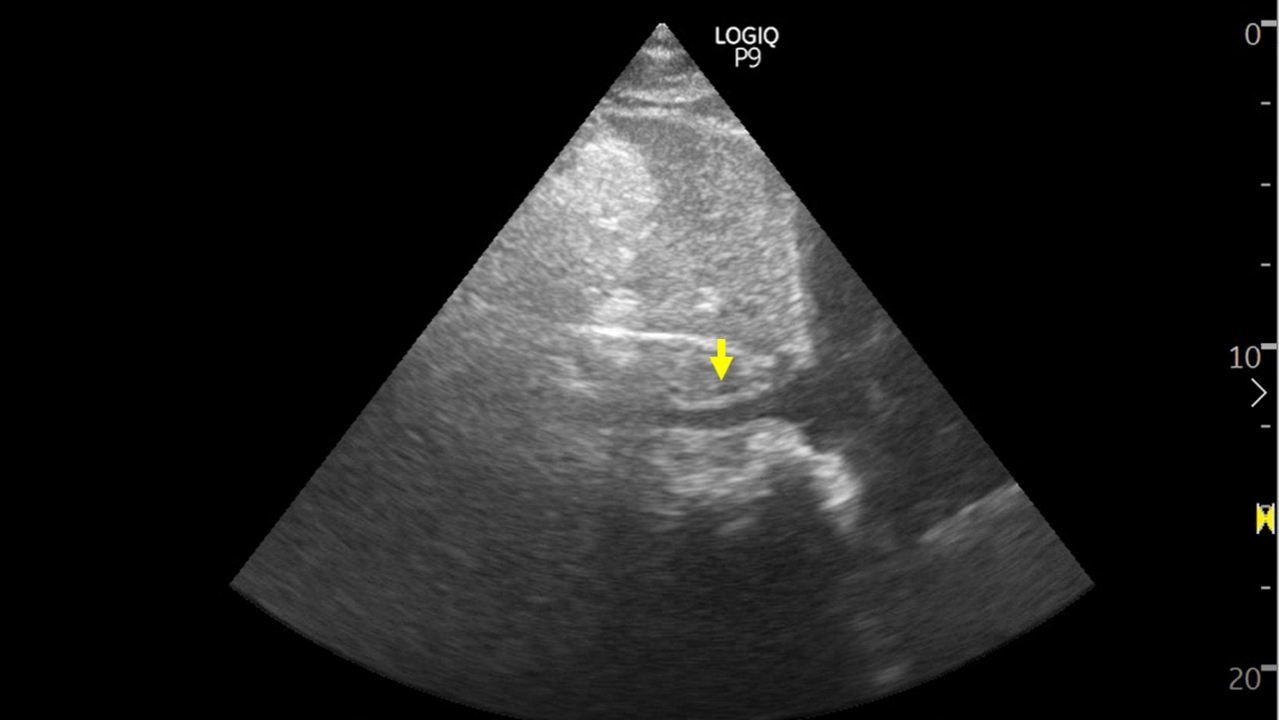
Sonographic image demonstrating a small inferior vena cava (maximal diameter). Online Video S1 demonstrates the respiratory variation. Image was obtained using a phased array transducer from the subxiphoid window with the probe orientation marker towards patient’s head.
